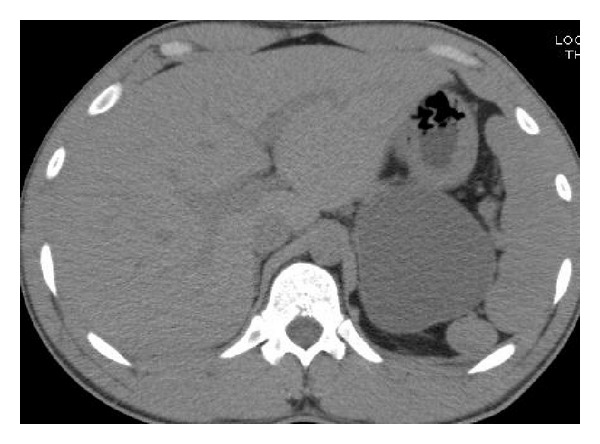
CT scan showing 8 × 7.4 × 6 cm homogenous, nonseptated cystic mass posterosuperior to pancreatic tail.
